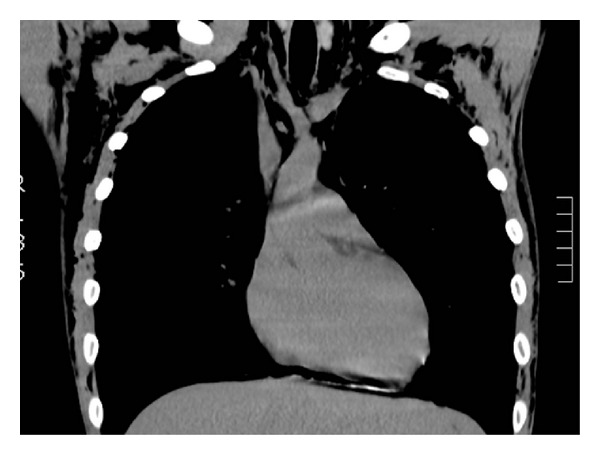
Coronal CT image showing pneumomediastinum and subcutaneous emphysema.
